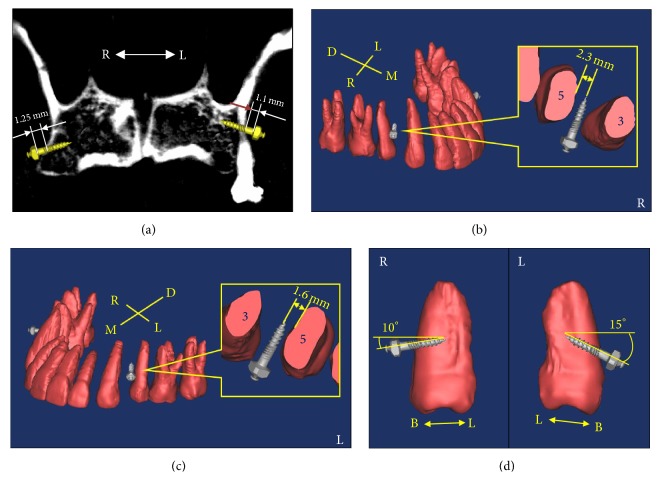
(a) Section image of CBCT reconstructed model to identify the contours of cortical, cancellous bone and teeth for planning the miniscrews microthreads to contact the cortical bone layer; (b) and (c) 3D solid model of the maxillary bone with teeth was reconstructed for surgical miniscrew planning at right and left sites; (d) two miniscrews were planned to insert to the occlusal surface inclination at angles of 15° and 10° for the left and right placement sites.
